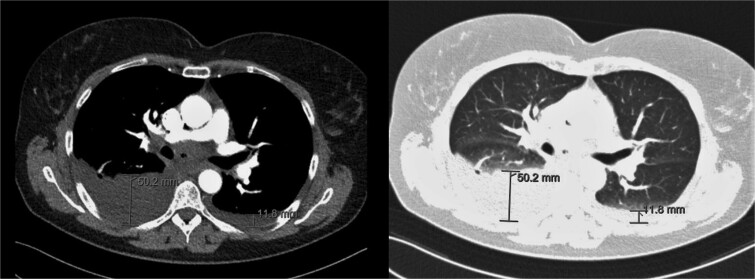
CT-scan during visit to the emergency department confirming the pleural effusion attributed as reactive or postoperative.
